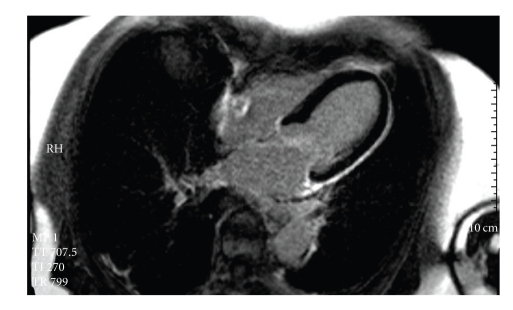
Cardiac magnetic resonance imaging showed a hyper-enhancement confined to the lateral wall.
